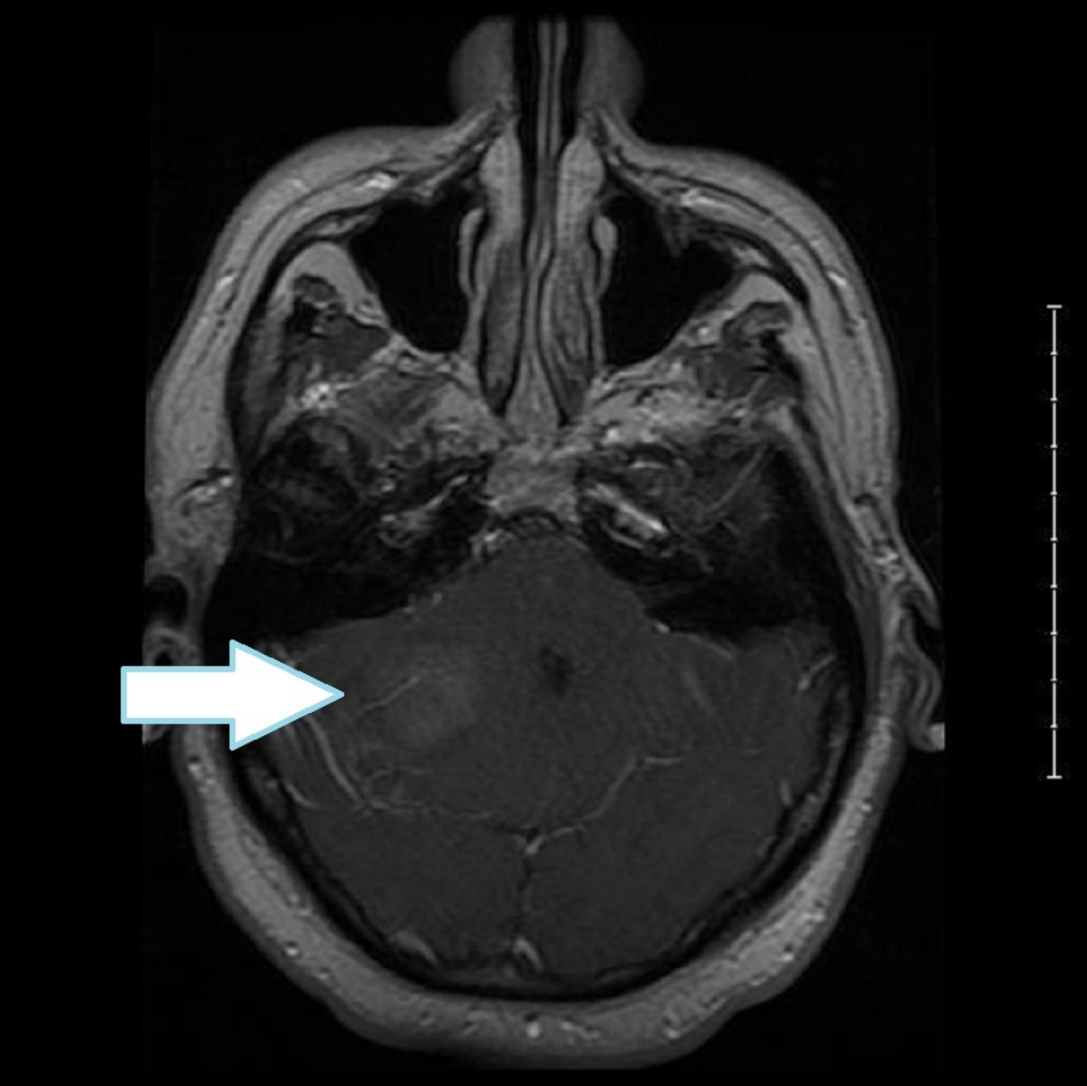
Preoperative MRI of brain with contrast post-axial T1 showing a hyper-dense posterior fossa tumor on the right side with mass effect and obstructive hydrocephalus MRI: magnetic resonance imaging
